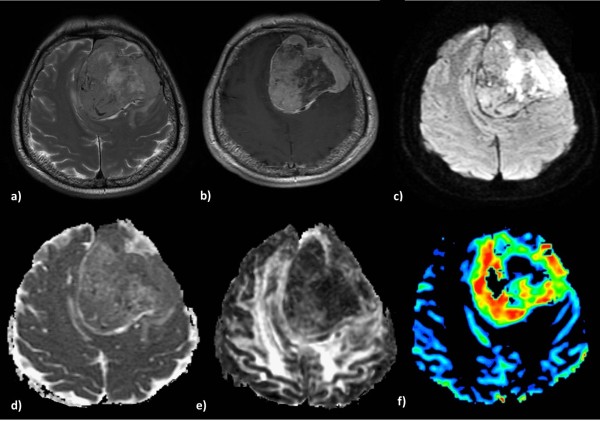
A typical Meningioma in a 60-year-old man. Axial T2-weighted (a) and postcontrast T1-weighted (b) images demonstrate a large heterogeneous enhanced left frontal mass with an intense mass effect. The lesion presents areas of restricted diffusion (c), isointensity on the ADC map (d), hypointensity on the FA map (e) and elevated blood volume (f).
